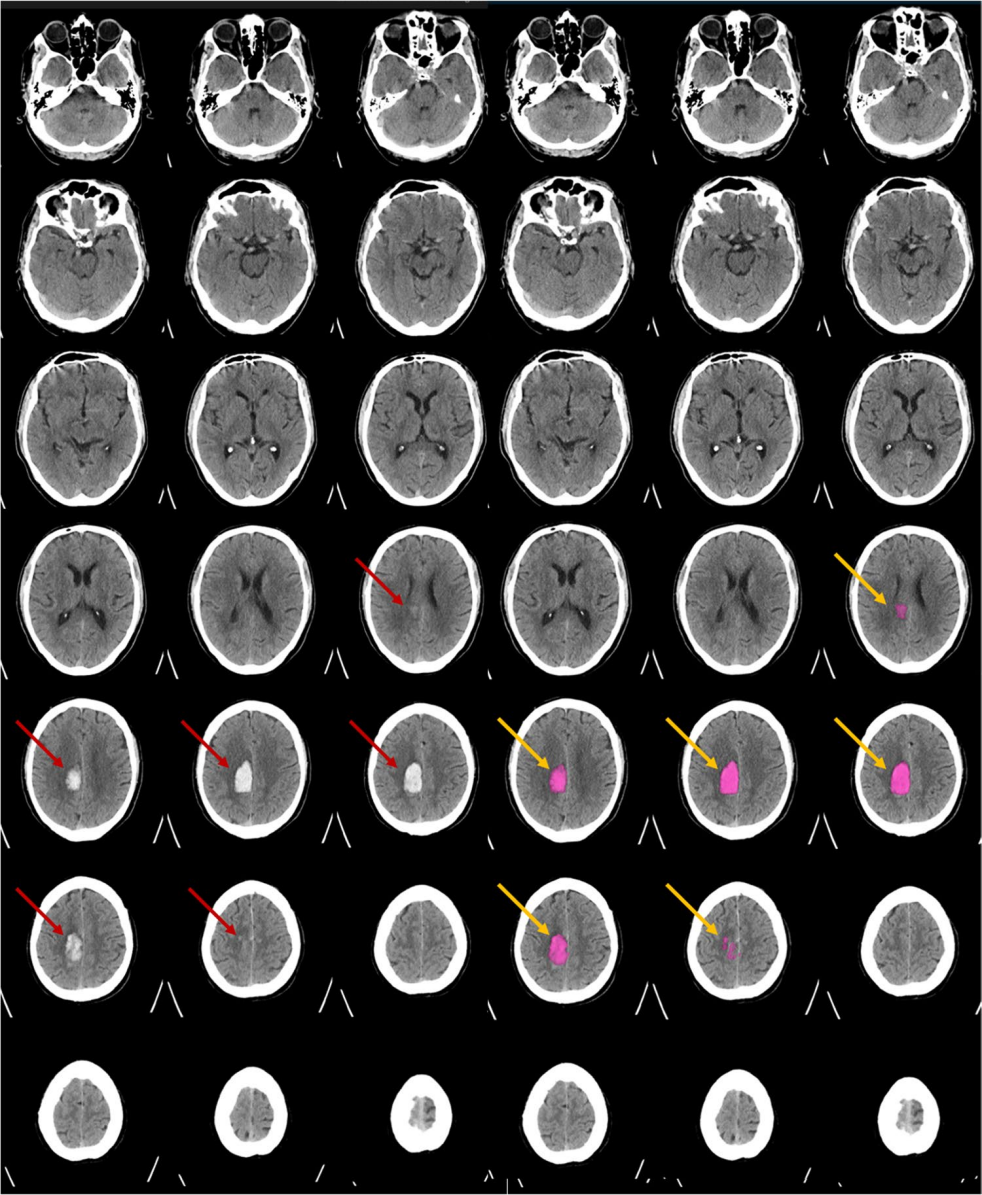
Example of a patient with acute stroke symptoms and intraparenchymal hemorrhage (IPH) on non-contrast-enhanced head CT (left, red arrows). The detection of IPH, segmentation, and volume quantification were performed automatically by the algorithm from Brainomix (right, yellow arrows) in nearly real-time. The above-demonstrated findings will be provided to the user. The fully automated quantification of the IPH in this example resulted in 11 ml
